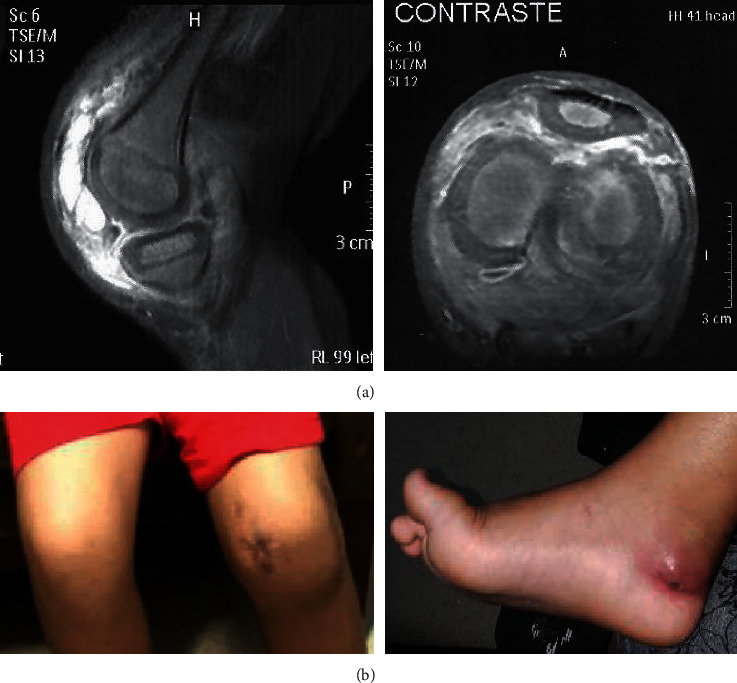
(a) Magnetic resonance imaging of the left knee. Sagittal plane (left); transverse plane (right), T2-weighted contrast. Presence of an extraarticular infiltrative process with involvement of the subcutaneous tissue and epidermis; inflammatory infiltration of adjacent muscle tissue, without evident collections; small foci of inflammatory edema affecting the subcortical bone marrow; extensive synovitis in the patellar recess and intraarticular involvement. In the transverse plane, there is an increase in the inflammatory process, with volumetric impingement of the popliteus neurovascular bundle. (b) Clinical characteristics of the pyoarthritis. Sequelae with increased left knee volume (left); the inflammatory process in the right ankle caused by trauma from the buckle on footwear (right).
